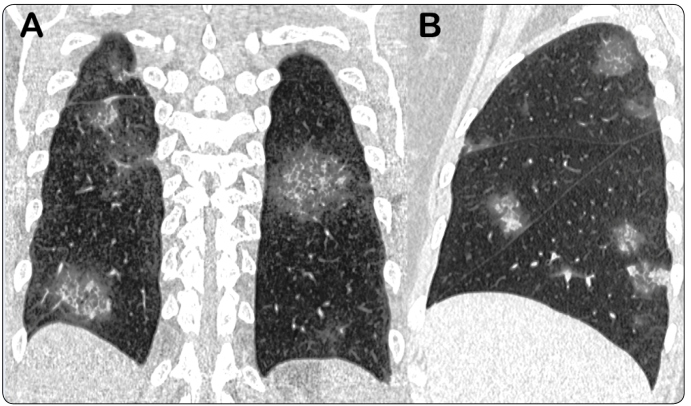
(A) Coronal reformatted CT image of a 59-year-old man with COVID-19 presenting with fever and showing multiple bilateral rounded opacities with crazy-paving aspect. (B) Sagittal reconstructed chest CT image of a 52-year-old woman with COVID-19 pneumonia showing multifocal, patchy, rounded ground-glass and mixed opacities.
